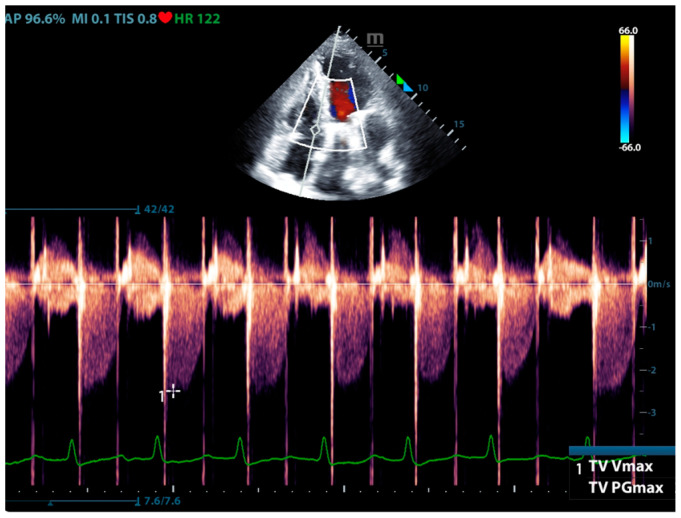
The ultrasound measurement of the maximum velocity of tricuspid valve regurgitation.
